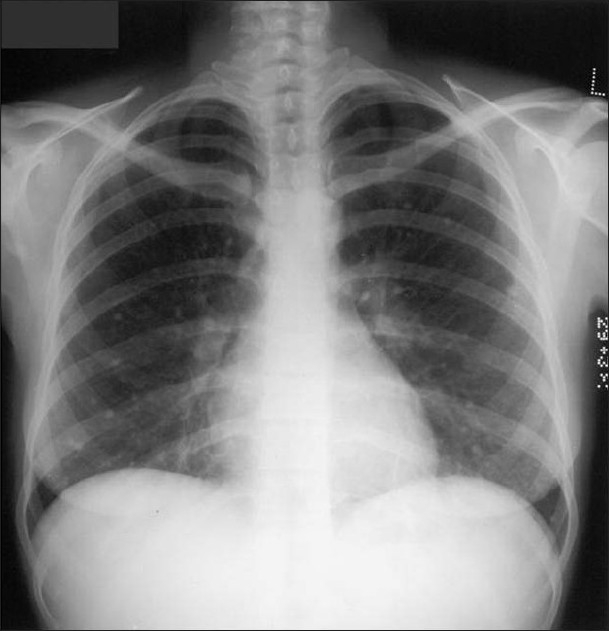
A chest radiograph shows multiple small calcific nodules in an adult female with a past history of Varicella pneumonia
